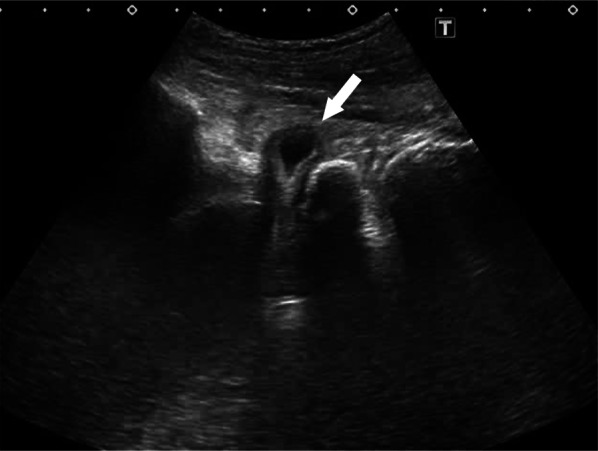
Percutaneous ultrasonographic image of incarcerated obturator hernia. The forward part of small intestine (white arrow)
